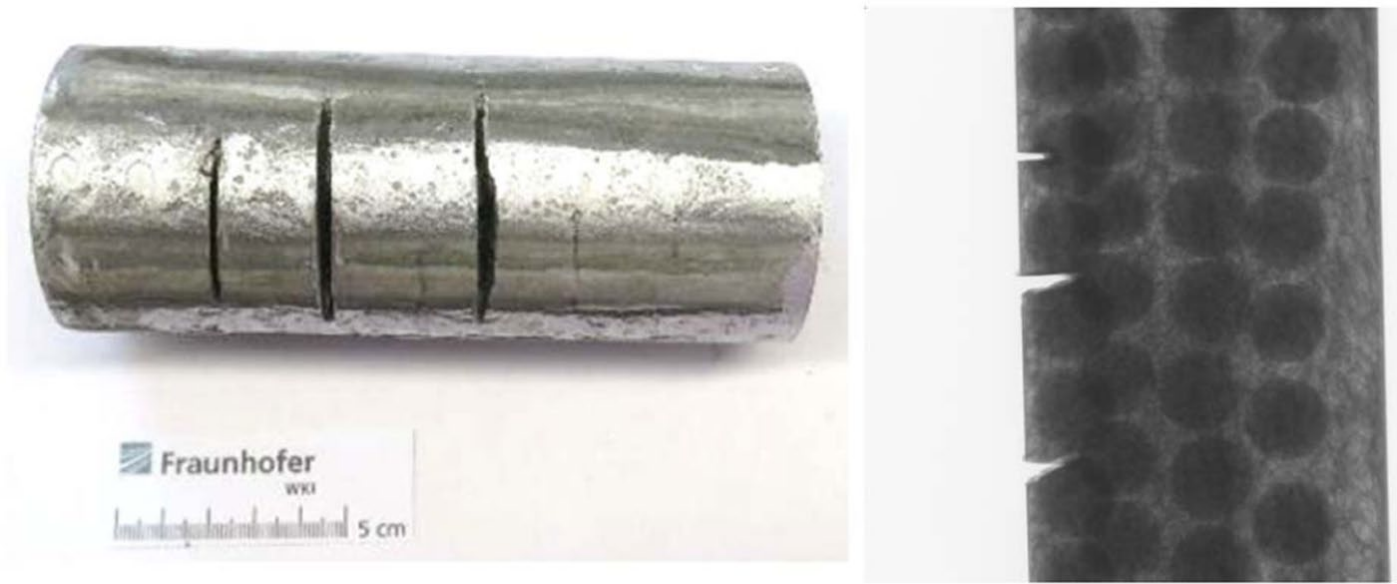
Cylindrical material sample after angle grinder cutting attack accompanied by an x-ray transmission image. A series of transmission images is the basis of CT measurements.
